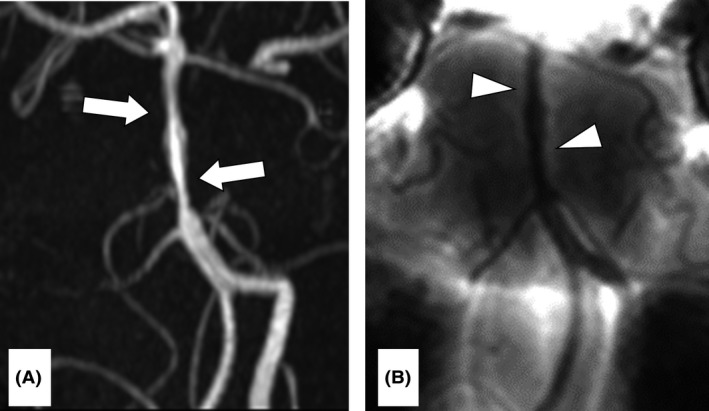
Initial imaging in the emergency department of a 41‐year‐old man with reversible cerebral vasoconstriction syndrome of the basilar artery. A, Magnetic resonance angiography of the brain showing multifocal segmental narrowing of the basilar artery (arrows). B, Basi‐parallel anatomical scanning–magnetic resonance imaging showing a multifocal narrowing vascular lesion with the same appearance as on magnetic resonance angiography.
